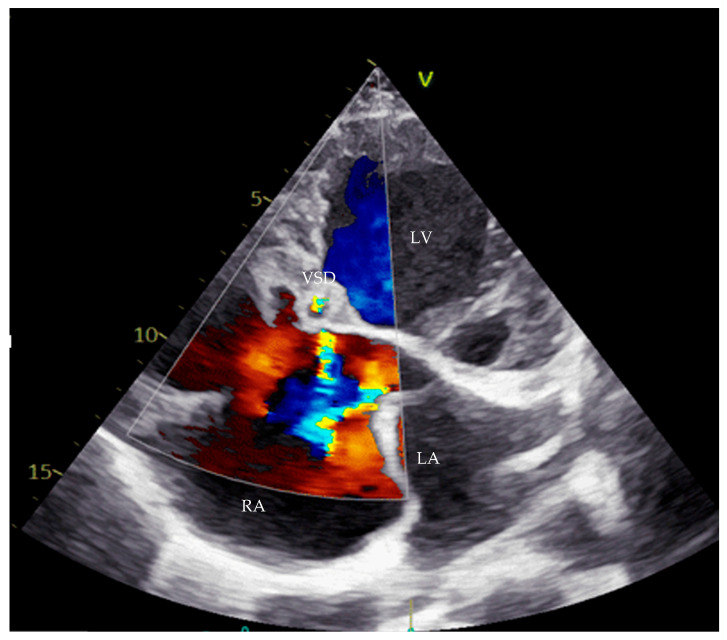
Echocardiography with apical four-chamber view showing VSD Gerbode type. LV—left ventricle, LA—left atrium, RA—right atrium, VSD—ventricular septal defect.
